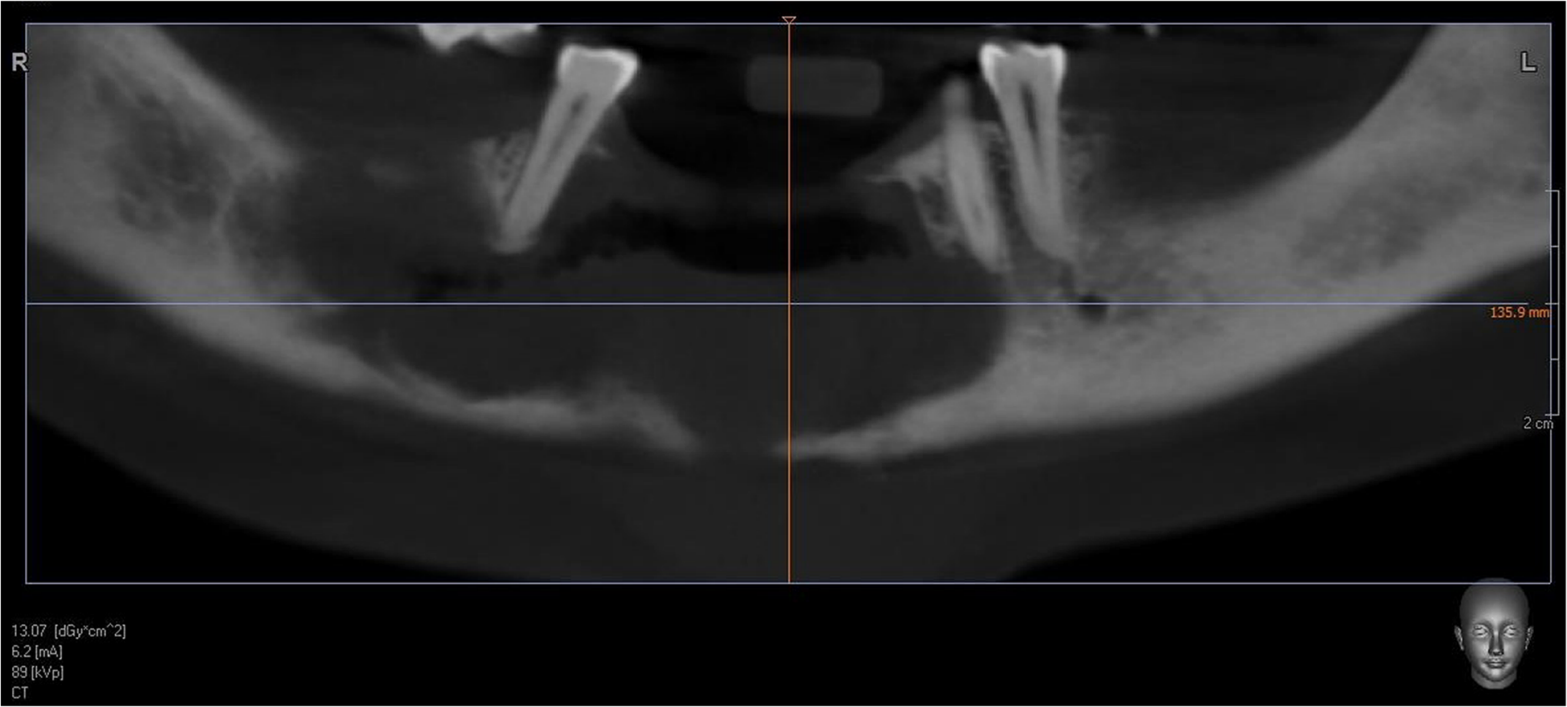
Cone beam computed tomography of the mandible. Pseudo-dental pantomogram view of lesion
